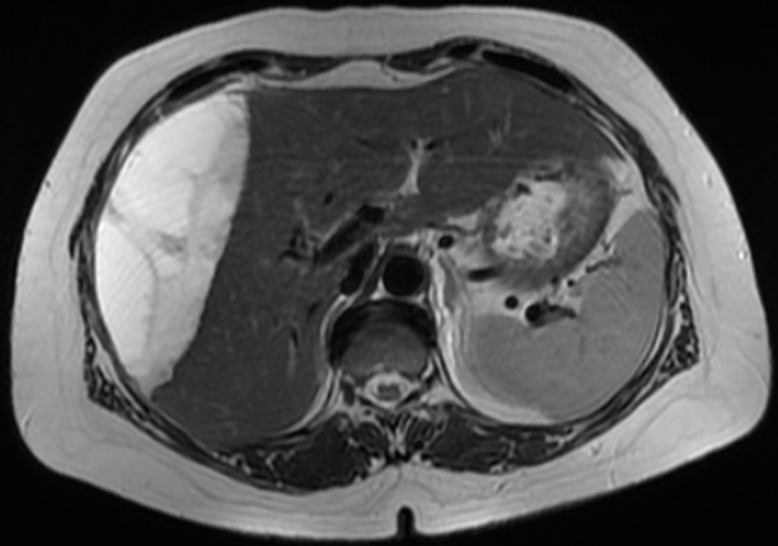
MRI showing giant sub-capsular hematoma of the liver without any traumatic or hepatic tumour pathology
